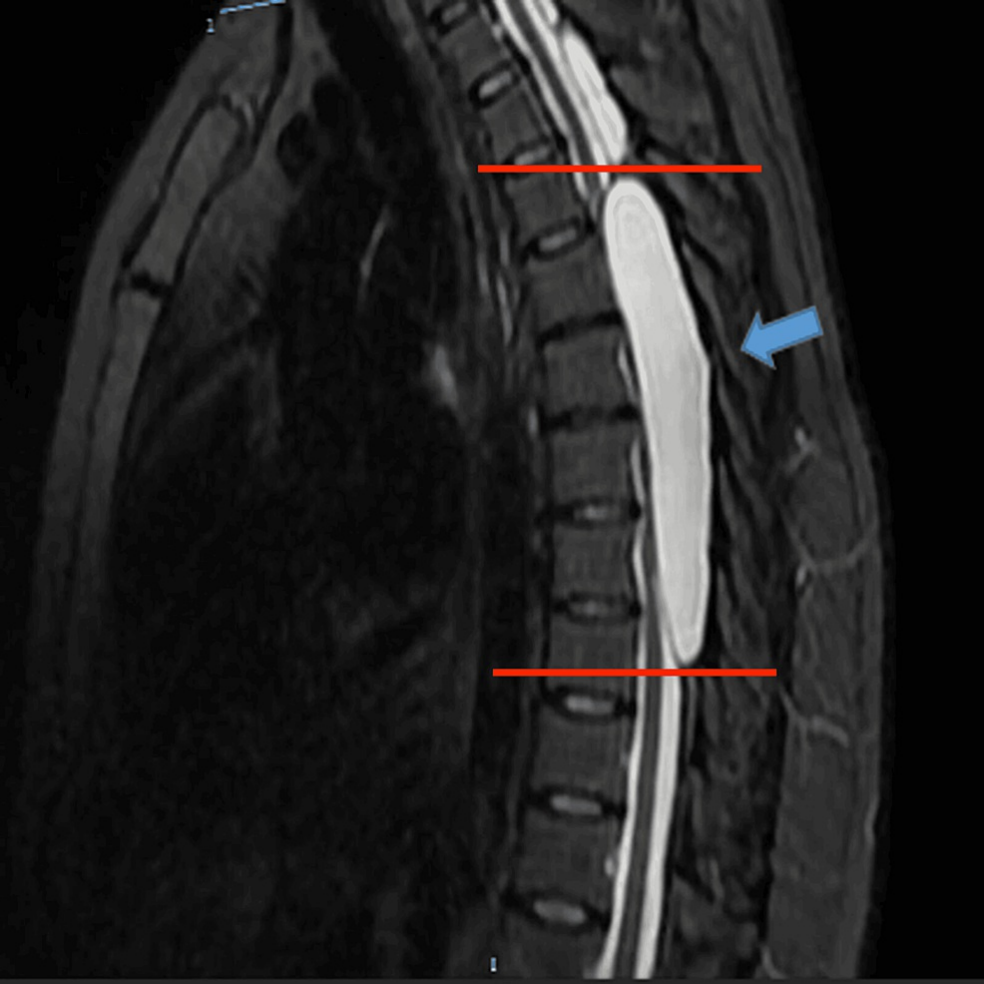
Preoperative imaging study The T2 MRI sequence sagittal section shows a hyperintense epidural lesion (a blue arrow) from level T3 to T8 (marked with red lines), compressing the spinal cord from dorsal to ventral with defined borders. MRI: Magnetic resonance imaging.
